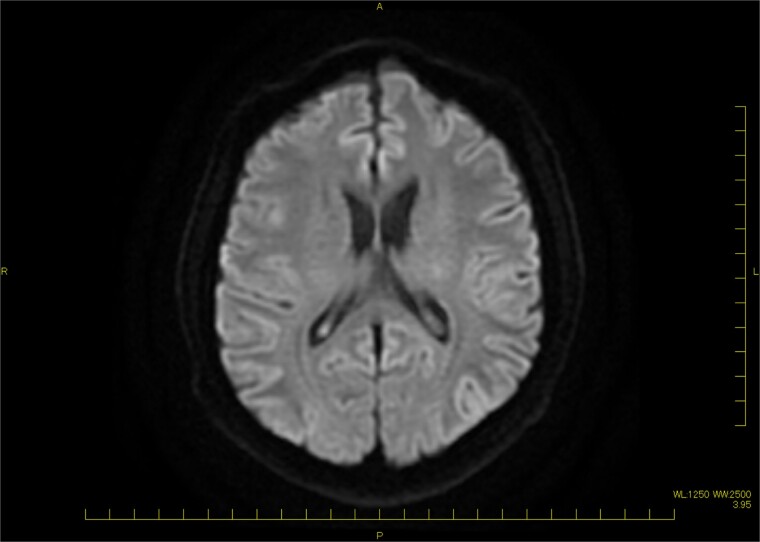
Brain MRI (DWI) showing no acute lesion.
